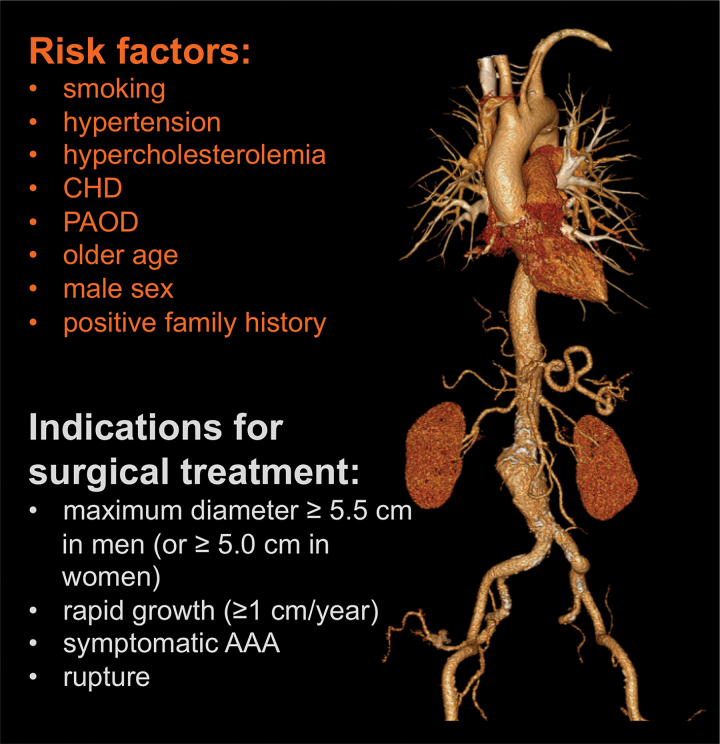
AAA in spotlight. Computed tomography angiogram of the thoracoabdominal arterial tree presenting with an infrarenal AAA. Presence of concomitant atherosclerotic calcifications predominantly in the regions of the aortic neck and the iliac arteries is frequent and attributable to similar risk factors. AAA, abdominal aortic aneurysm; CHD, coronary heart disease; PAOD, peripheral arterial occlusive disease. Color images are available online.
